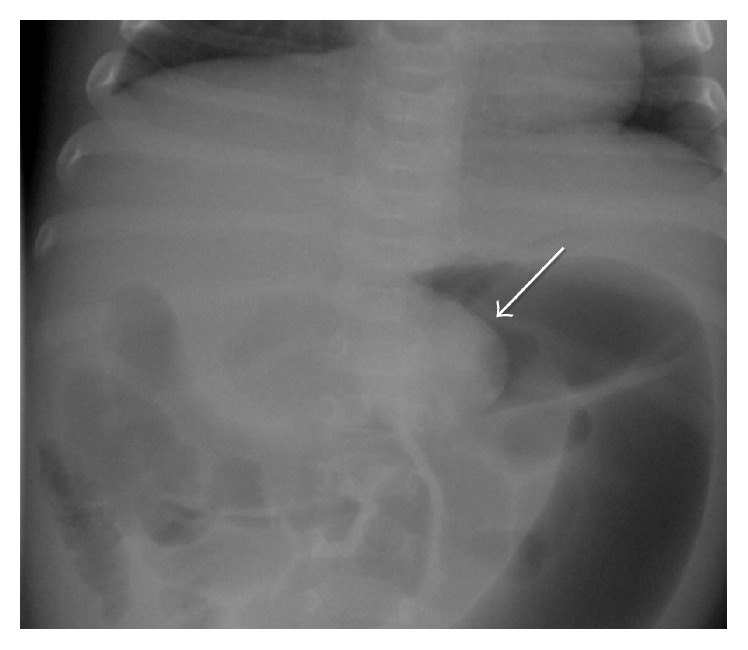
Abdominal radiograph after subsequent air enema demonstrates a “meniscus sign” of intussusception.
